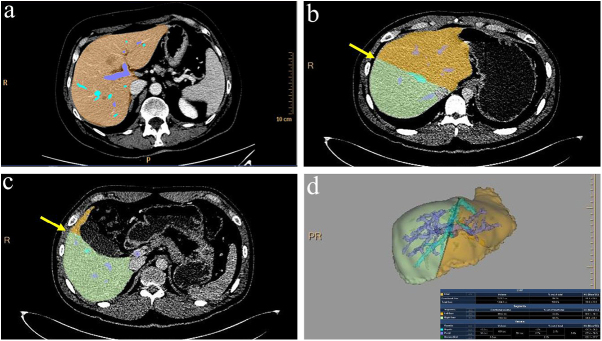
Automated volumetry of the liver by Philips Healthcare. (a) The liver was segmented on axial CT images, and the total liver volume was automatically computed. The system detected vascular structures, classifying them as portal, hepatic, or unclassified vessels, which were included in the volume analysis. (b) A radiologist defined the planned resection plane to the right of the middle hepatic vein (arrow). (c) The radiologist delineated the resection plane starting from the gallbladder bed (arrow). (d) The artificial intelligence (AI) algorithm automatically propagated the defined resection planes and mapped the major vascular structures to enhance surgical planning.
